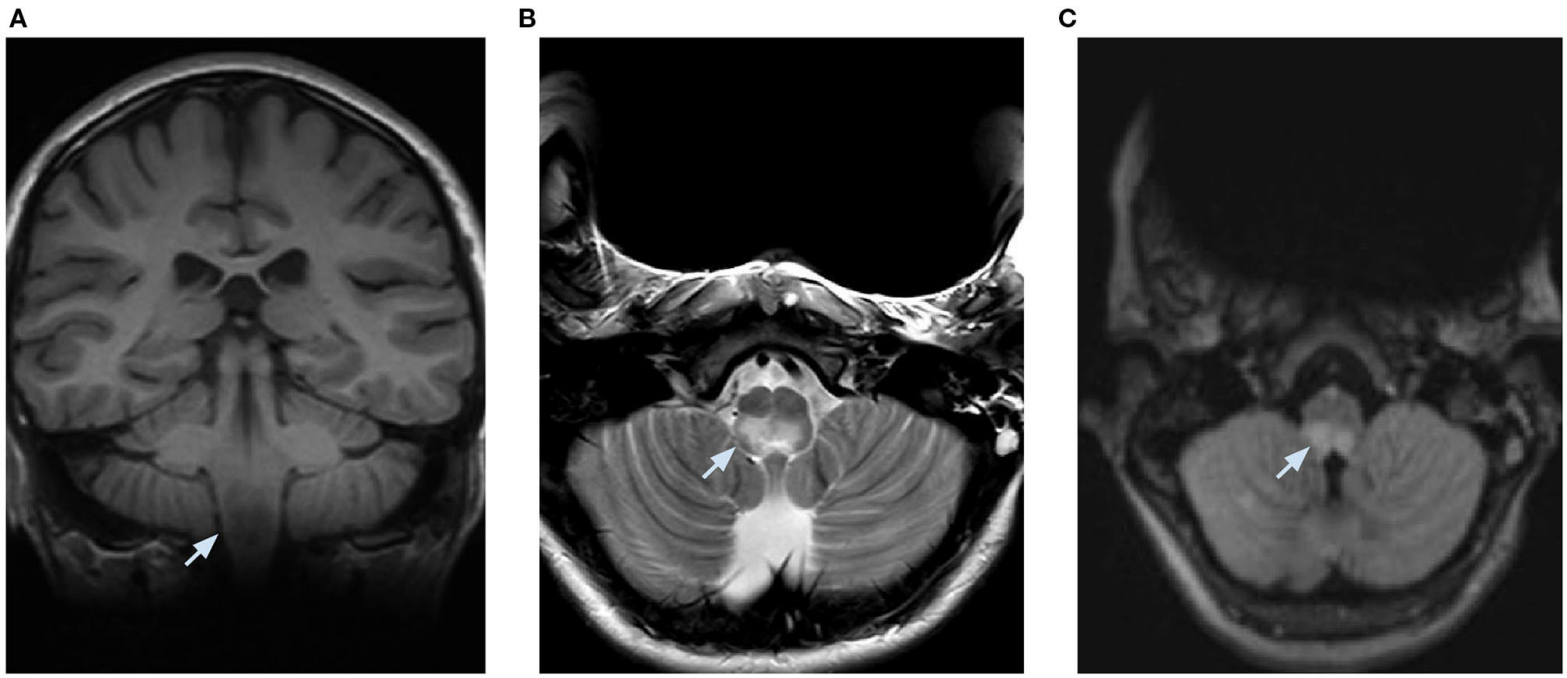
Brain MRI performed at 1.5 Tesla and limited by metallic artifacts. (A) coronal T1 weighted imaging showing a low signal intensity, (B) axial T2 weighted imaging, and (C) axial fluid attenuation inversion recovery showing a high signal intensity in the posterior part of the medulla oblongata bilaterally.
